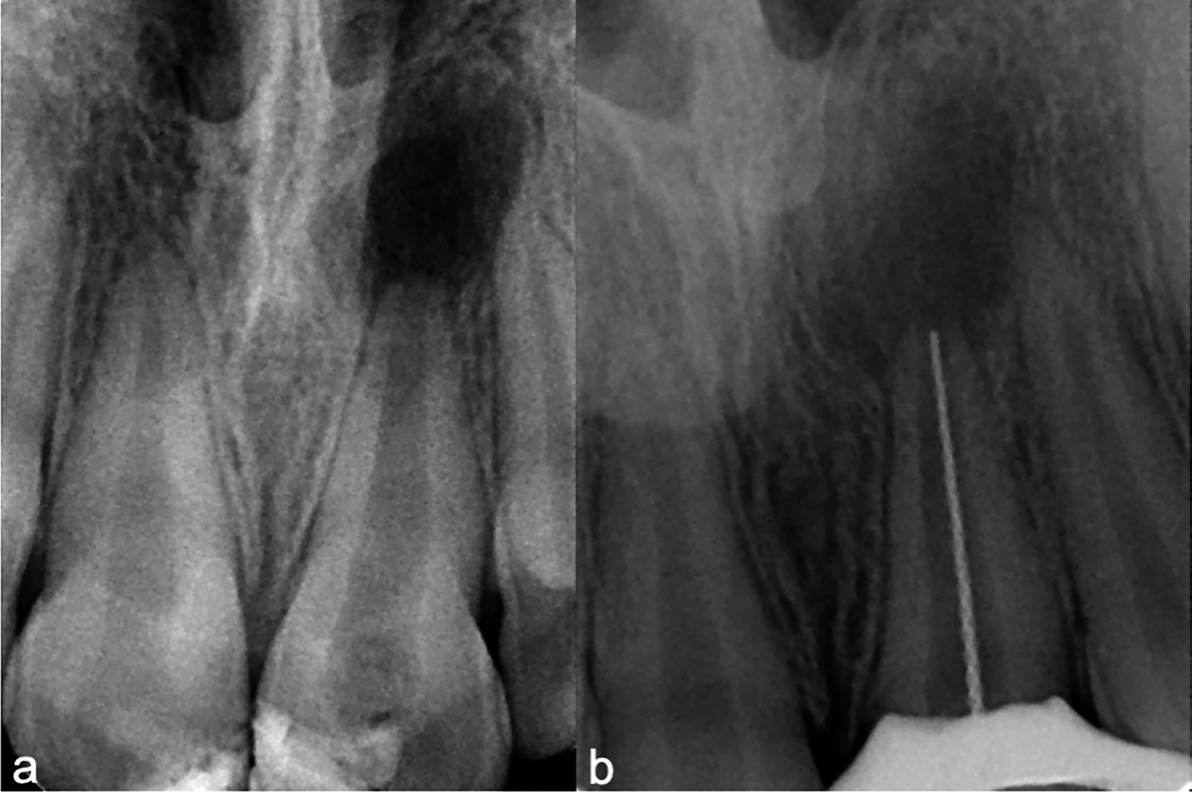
Baseline and intraoperative periapical radiographs of tooth 21. (a) Preoperative periapical radiograph showing an immature apex (Nolla stage 9) and a well-defined apical radiolucency consistent with asymptomatic apical periodontitis in an 11-year-old patient with a history of dental trauma. (b) Working length determination radiograph (21 mm) using a #40 K-file during canal disinfection and preparation prior to regenerative endodontic therapy.
